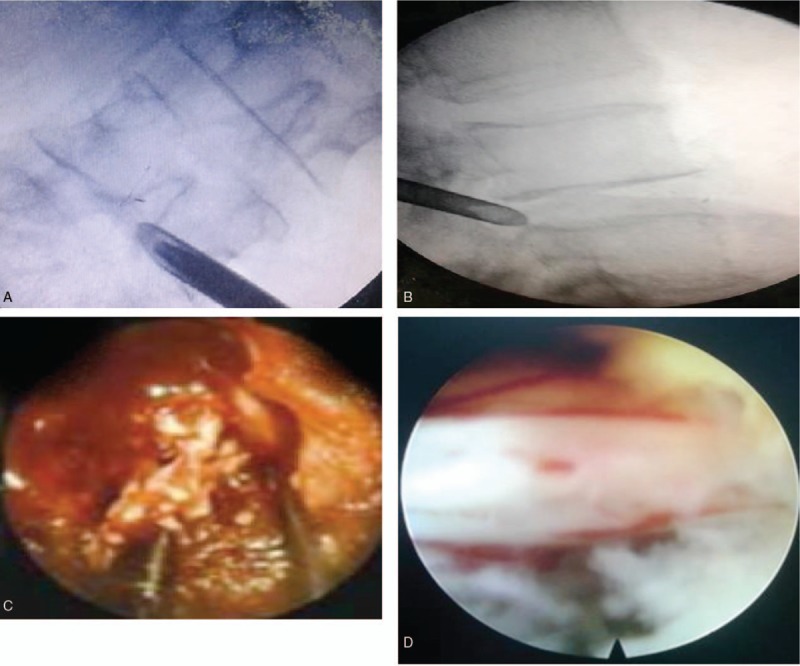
Instruments used for MED. (A) Image intensifier radiographs showing intraoperative level of disc herniation and insertion of serial dilators anterior-posterior view. (B) Radiographic image of serial dilator (lateral view). (C and D) The removal of a disc with the free release nerve root.
